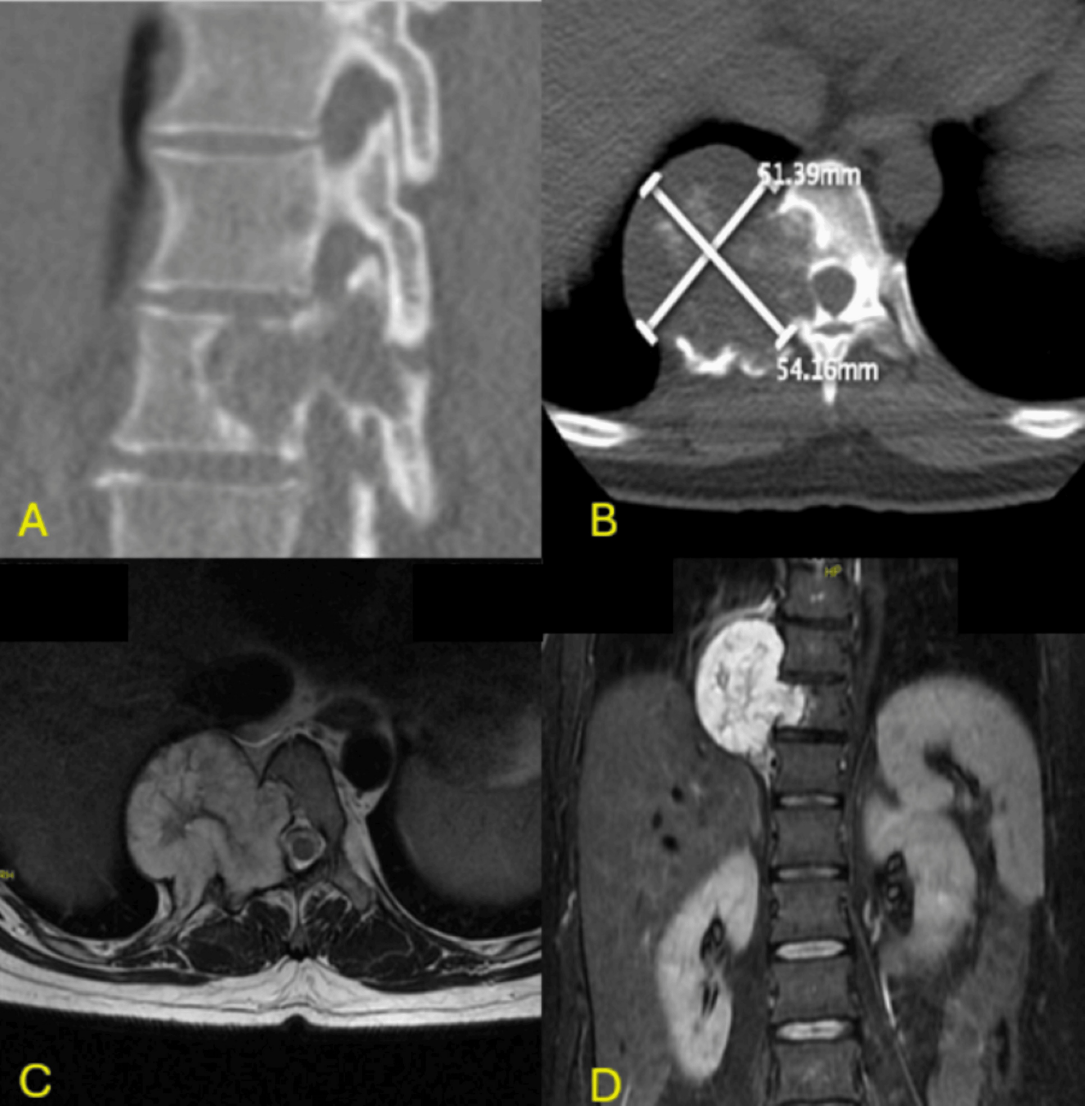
Radiographic images showing the chondrosarcoma pre-operatively 1A: CT sagittal view showing an osteolytic lesion involving the T10 vertebral body with extension to the posterior elements on the right side 1B: CT axial view showing an osteolytic lesion in the right half of the T10 vertebral body with extension to the pedicle and adjacent rib. 1C: MRI axial T2 image showing an expansive, lobulated lesion involving the right-sided posterior elements of the T10 vertebral body and the posterior aspect of the right tenth rib. The expansile lesion mildly effaces the right lateral margin of the spinal canal. 1D: MRI STIR coronal image showing an expansile lobulated lesion involving the right half of the T10 vertebral body. The lesion effaces the lateral aspect of the T9 and T11 bodies on the right side. STIR: short tau inversion recovery
